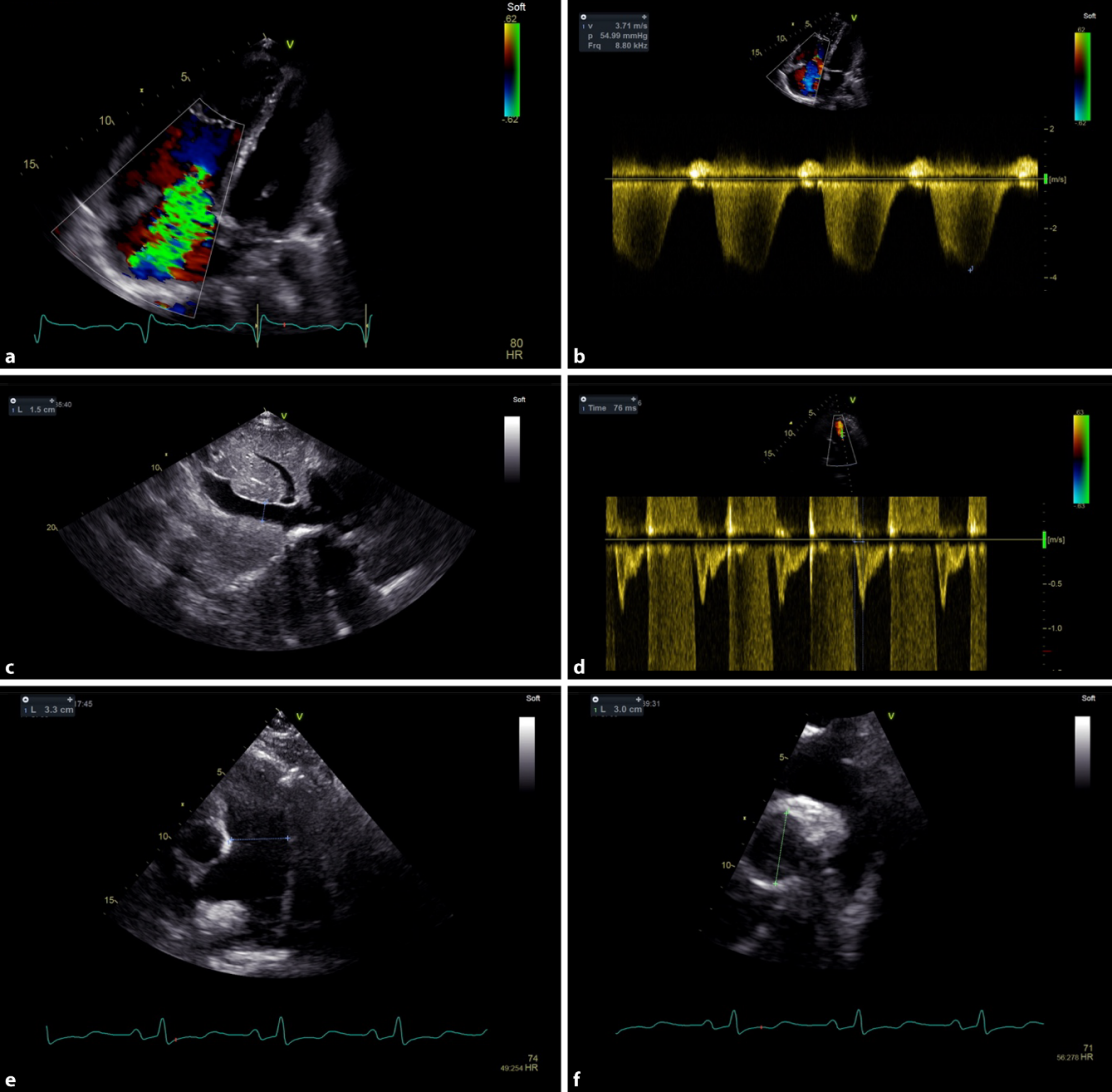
Systematic evaluation of signs of pulmonary hypertension. Maximum jet velocity over tricuspid regurgitation (panels a and b). Diameter and respiratory change of inferior vena cava (panel c). Pulmonary valve acceleration time (panel d). Dilatation of the pulmonary artery (panel e) showing the pulmonary trunk in the parasternal short axis and panel f showing the right pulmonary artery from suprasternal angulation
